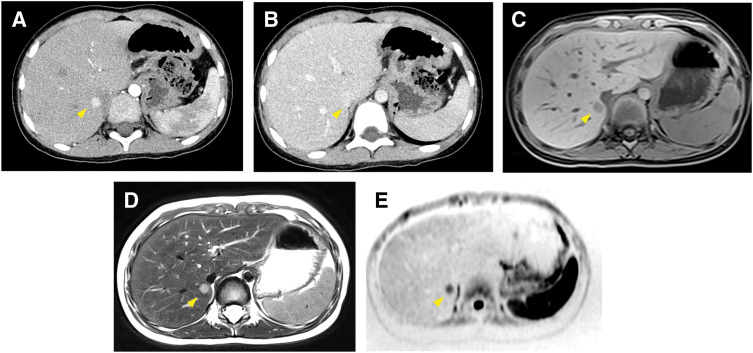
Imaging findings of the hepatic lesion. (A) Contrast-enhanced abdominal CT (arterial phase) showing a 10-mm hypervascular nodule in S1 (arrowhead). (B) Equilibrium phase demonstrating the nodule with contrast retention isodense to normal liver parenchyma (arrowhead). (C) T1-weighted MRI showing a hypointense nodule in S1 (arrowhead). (D) T2-weighted MRI demonstrating a hyperintense nodule in S1 (arrowhead). (E) Diffusion-weighted imaging revealing restricted diffusion within the lesion (arrowhead). S1, segment 1
